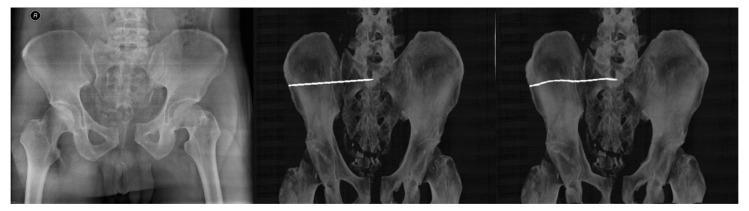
Result of Demons algorithm for multimodal image registration. (Left, Middle, Right): Target (reference) X-ray image, moving (input) DRR projection image, the output of image registration.
